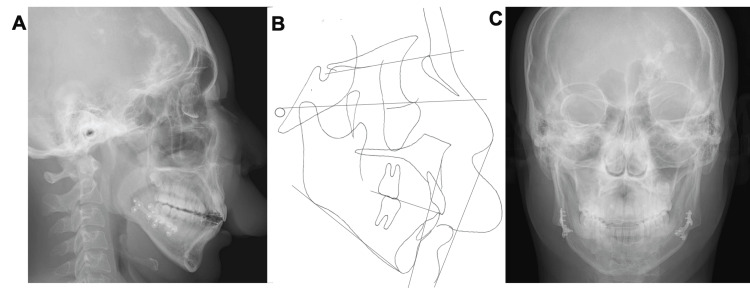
Post-treatment evaluation. (A) Lateral cephalometric radiograph. (B) Original tracing of lateral view. (C) Frontal cephalometric radiograph.
